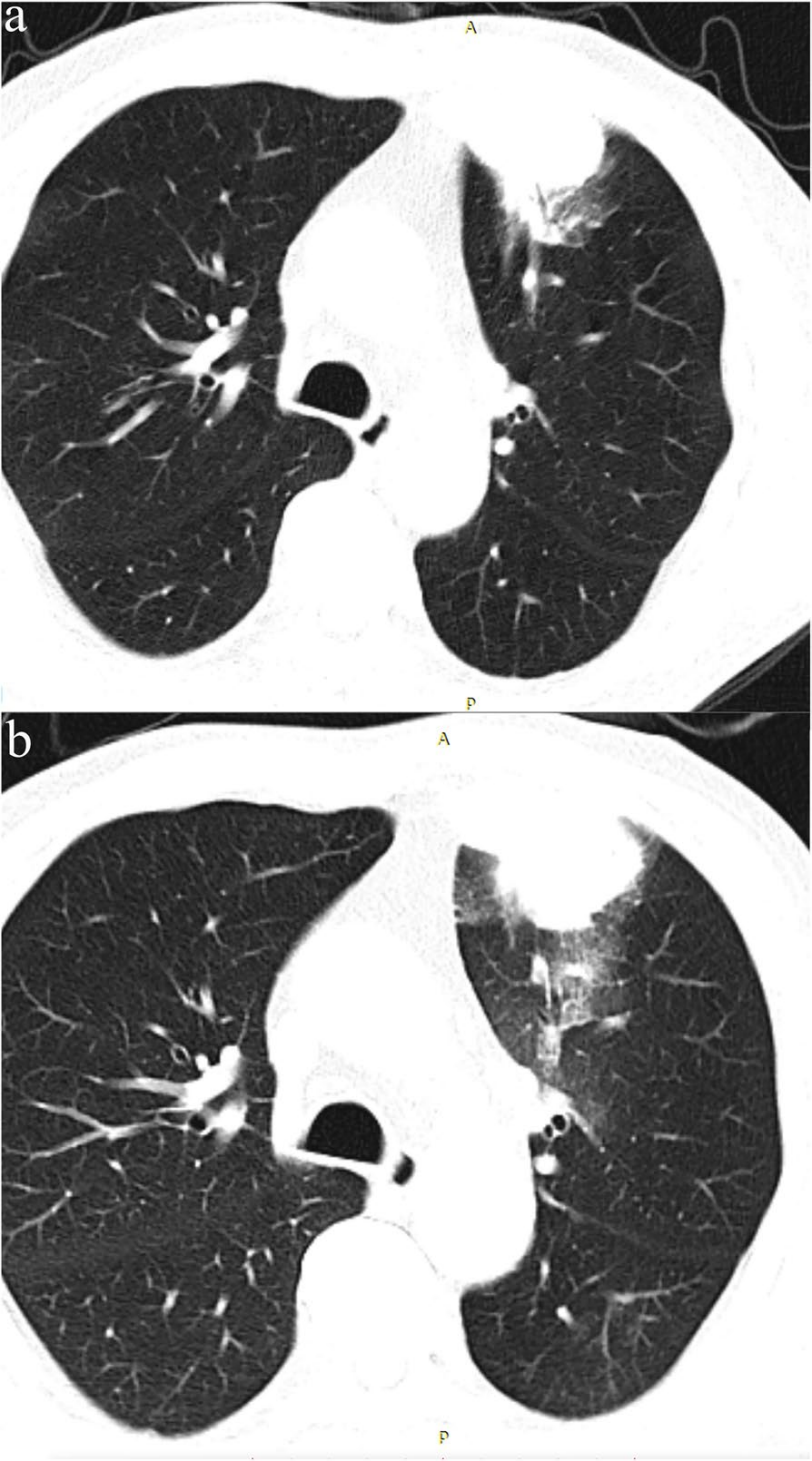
a Pre-procedure CT: A 69-year-old man with a 29-mm solid pulmonary lesion in the left lower lobe. b On the post-procedural CT scan, a ground-glass opacity was observed in the pulmonary parenchyma. However, the patient did not experience any episodes of hemoptysis
